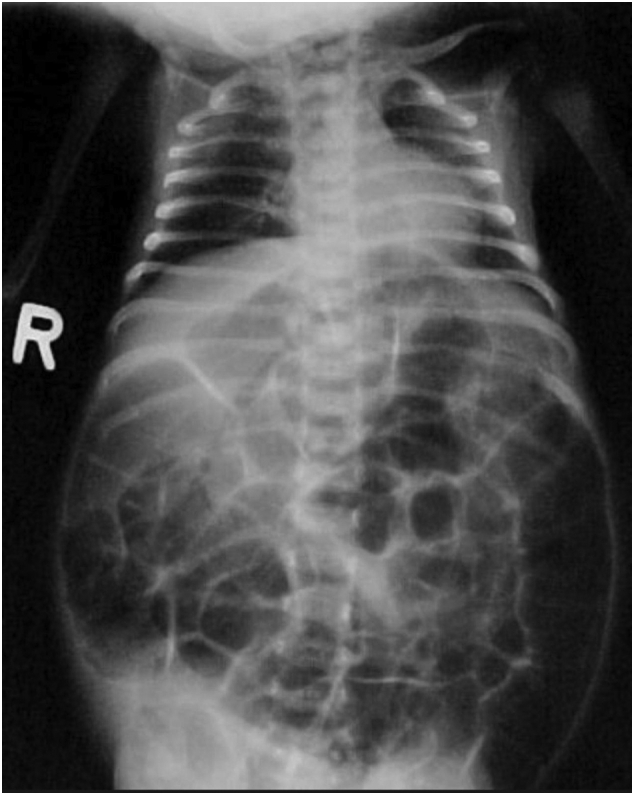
Plain abdominal X-ray supine view showing multiple loops of dilated large bowel suggesting large bowel obstruction.
